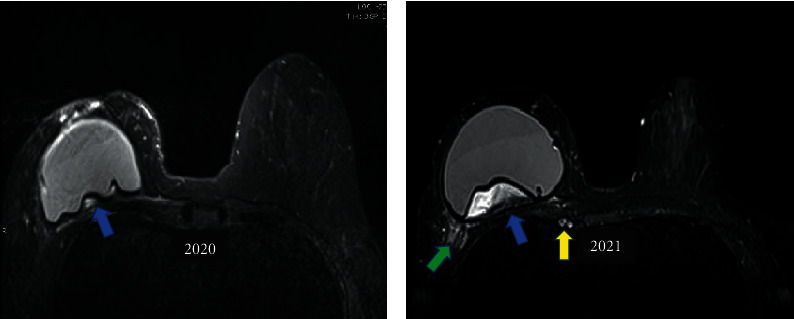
(a and b). Mass with a high signal on T2^*∗∗*^. Heterogeneous intracapsular tissue with a heterogeneous high signal is seen on the T2-weighted sequence^*∗∗*^ (blue arrow). Follow-up of silicone granuloma, 2020 (a) and 2021 (b). In the evolutive control, signs of pericapsular inflammation are also observed (green arrow).
